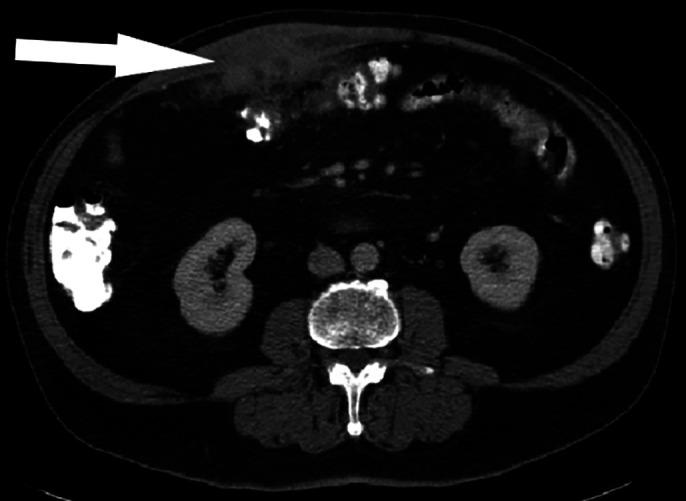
Abdominal and pelvic computed tomography with contrast 6 months after initial presentation showing increased size of soft-tissue density with surrounding fat stranding within the right medial rectus measuring 6.2 × 2.2 cm (white arrow).
